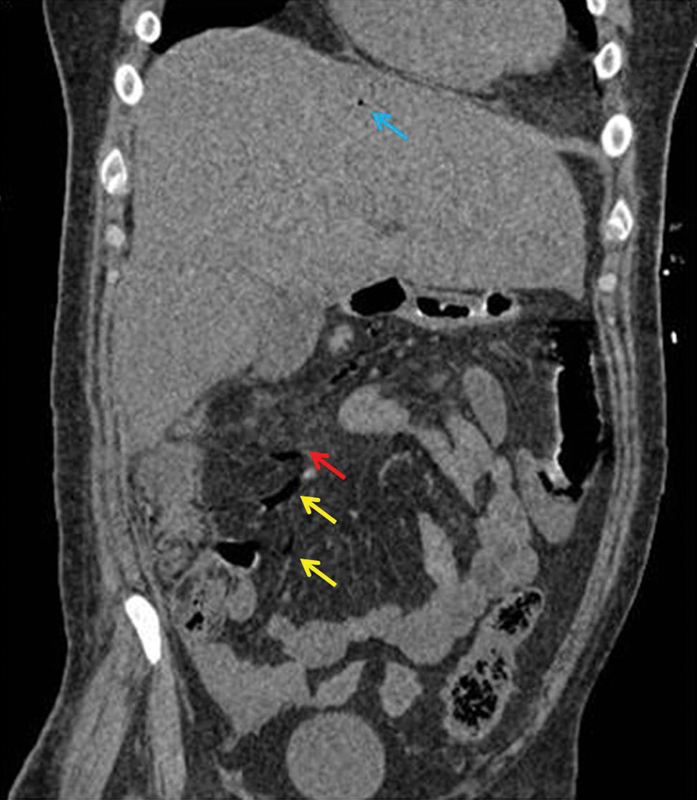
Coronal noncontrast computed tomography (CT) scan image showing air in the ileocolic vein (
